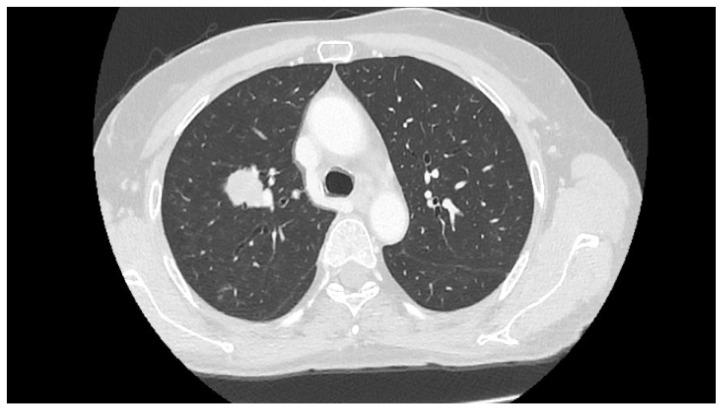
CT imaging on September 2020. Progression of disease with increase in size of the lung mass.
